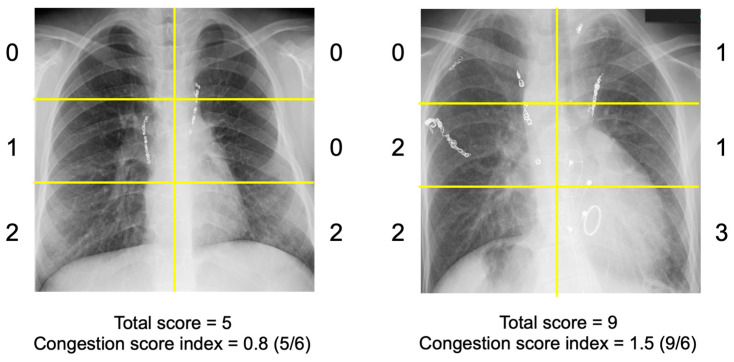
How to measure the CSI of a chest X-ray. Congestion scoring was conducted for each of the six lung field segments to provide a comprehensive assessment of pulmonary congestion. The grades of congestion were defined as follows: 0 = normal, 1 = cephalization, perihilar haze, peribronchial cuffing, or Kerley lines; 2 = interstitial pulmonary edema and localized or confluent mild edema; 3 = confluent intense edema. The total congestion score was calculated by summing the individual scores for all six lung segments. In cases where a lung segment was obscured or completely covered by pleural effusion, that segment was excluded from the scoring process to ensure the accurate representation of lung field conditions. To standardize the assessment across individuals with varying numbers of evaluable segments, the congestion score index was derived. This index was calculated by dividing the total congestion score by the number of lung segments that were available for evaluation, providing a normalized metric for the extent of pulmonary congestion.
